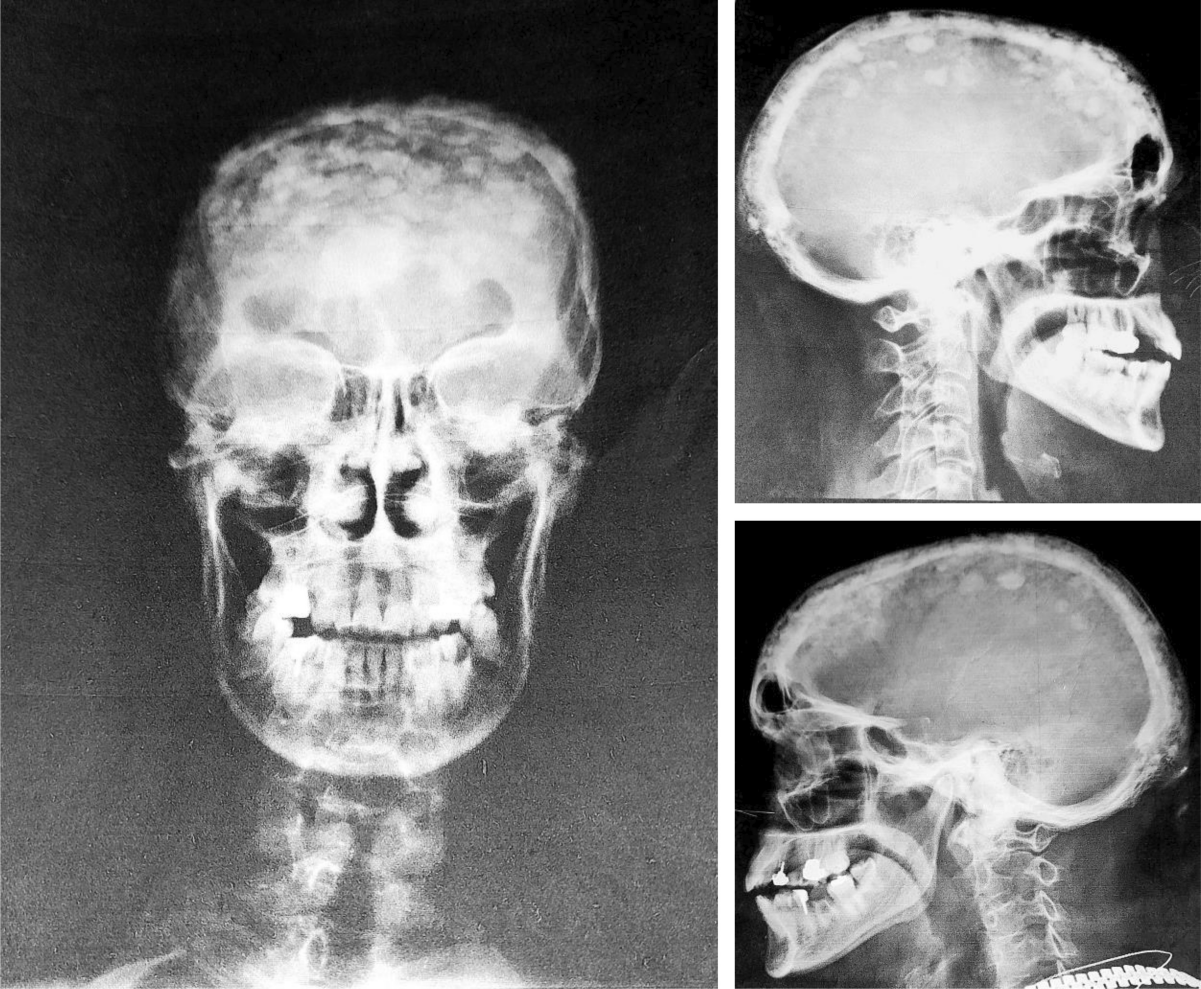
Plain radiography of skull showing poorly defined and fluffy sclerotic patches along with lytic lesions known as cotton wool appearance a classic sign of Paget's disease of bone.
